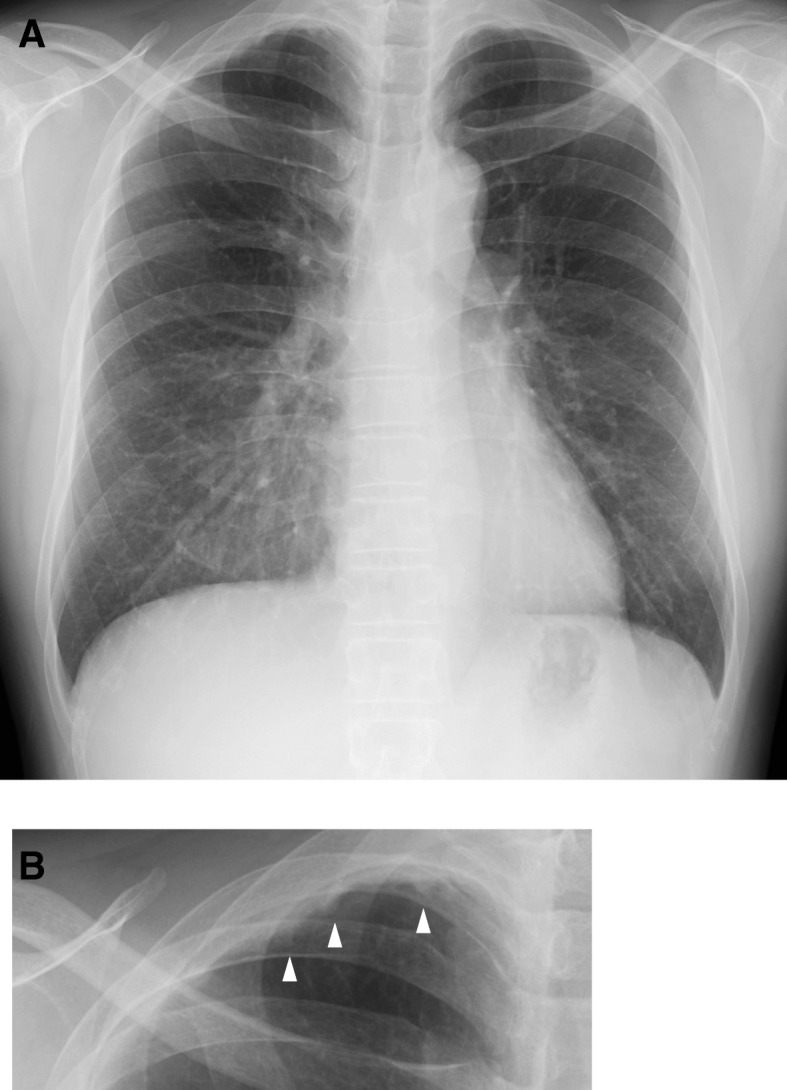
Representative chest X-ray image of pleural thickening. a. Chest X-ray image of a healthy 47-year-old male. The right apical cap appears as an irregular, wedge-shaped density. b. Enlarged image of the right apex. The white triangles indicate pleural thickening
